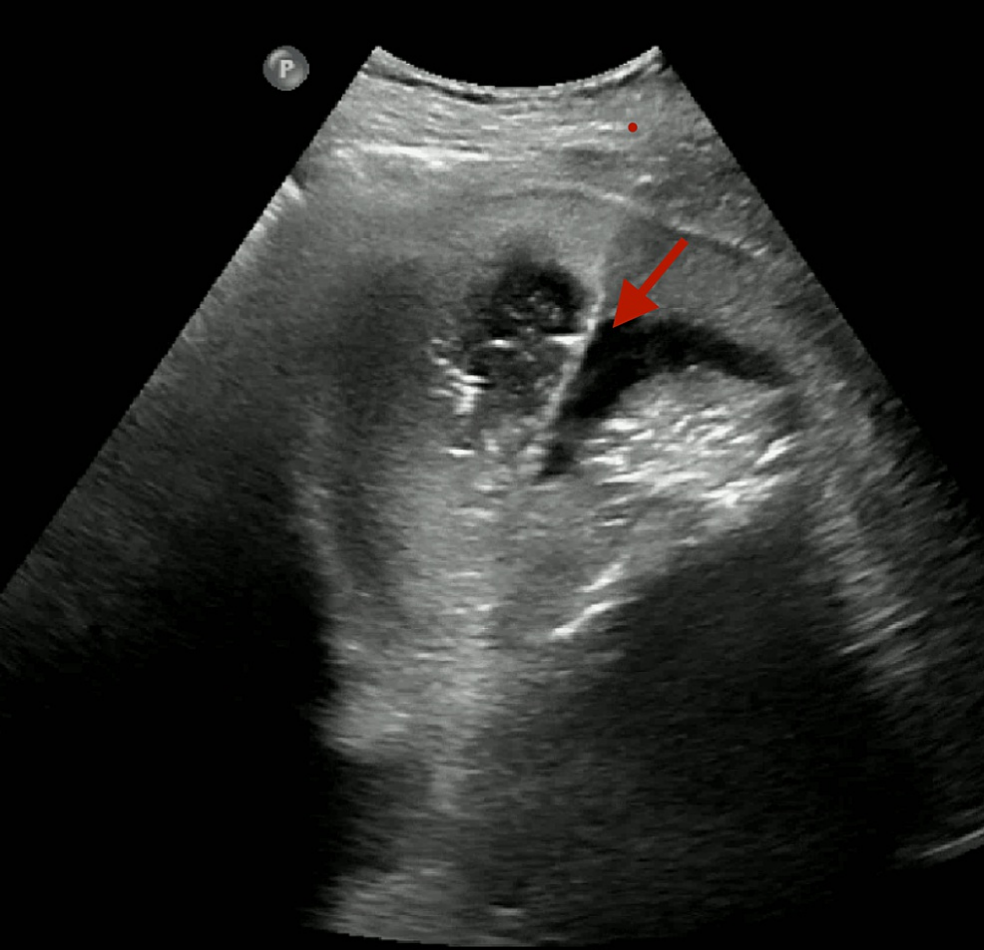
Ultrasound-guided percutaneous drainage of the air/fluid collection (performed within 24 hours of admission).
